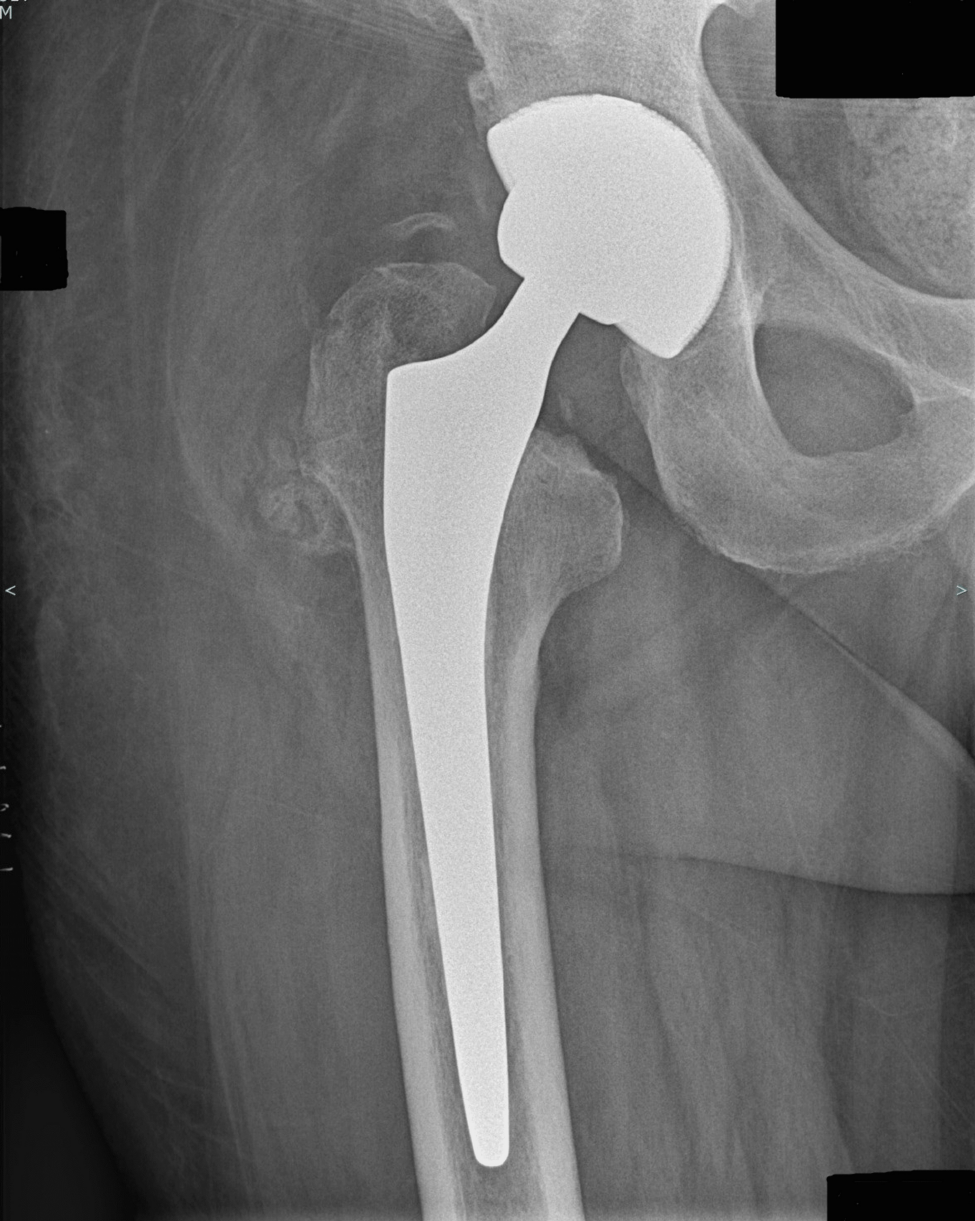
Radiograph of the revision arthroplasty showing the new hip prosthesis after the second stage of surgery.
